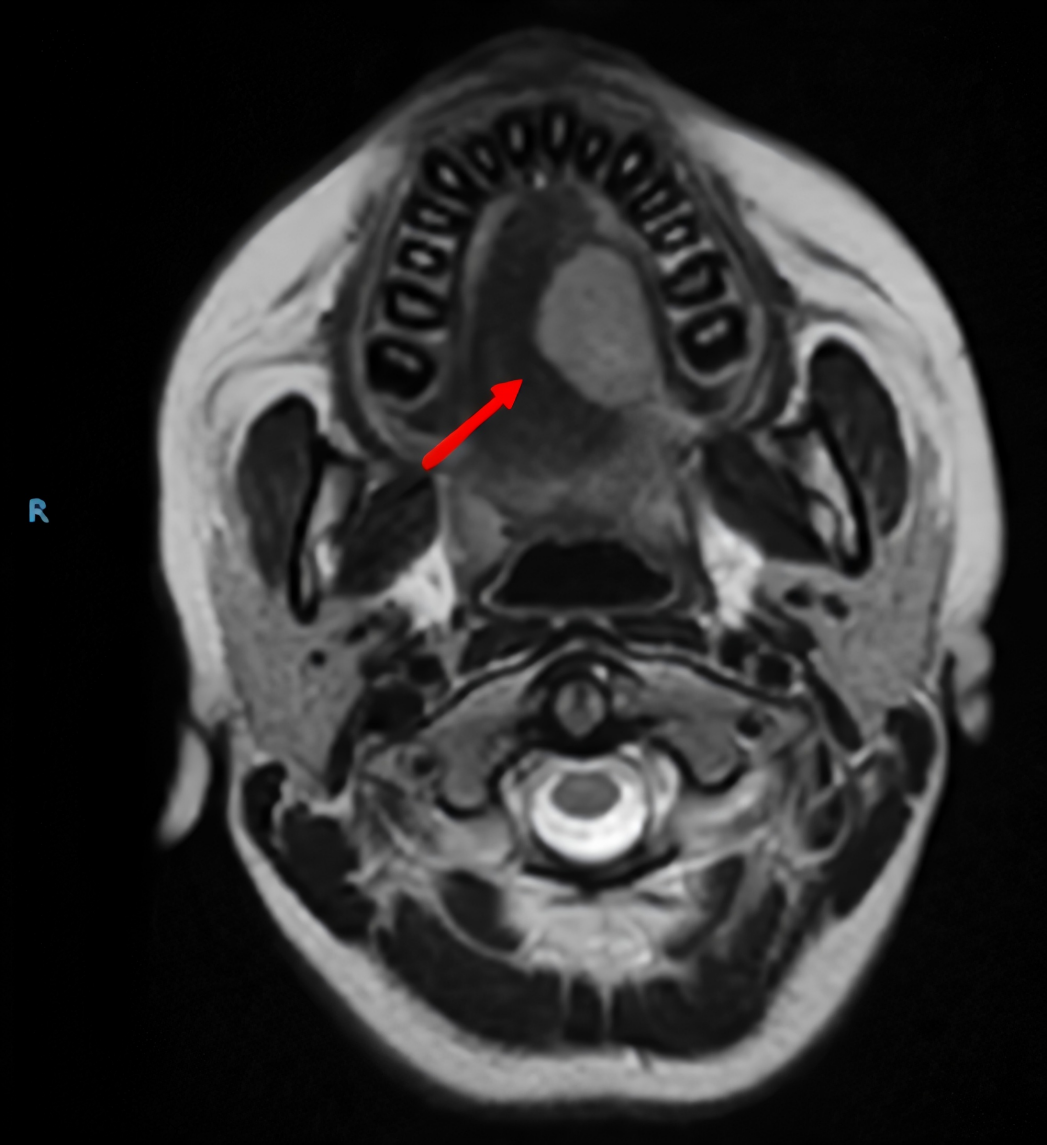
Magnetic resonance imaging of the face and neck. Involvement of the mucosa and the superior longitudinal, vertical, and transverse muscles of the lateral dorsum of the left hemilanguage was observed by a mass of well-defined and regular contours with increased intensity of signal in the T2-weighted sequences, with intense and homogeneous enhancement with the contrast medium, with measurements of 21 mm x 26 mm x 21 mm.
